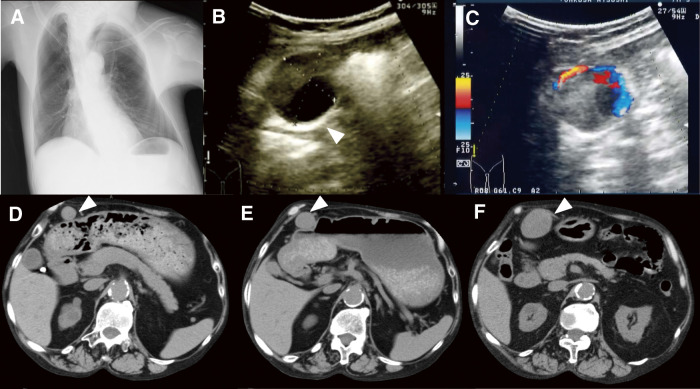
Fig. 1 Image findings. A chest X-ray revealed a scoliosis deformity (A). A sagittal sonogram identified a large anechoic mass (arrowhead) in the right hypochondrium (B). Color Doppler sonography demonstrated blood flow within the mass (C). Computed tomography imaging showed progressive enlargement of the gastroepiploic artery aneurysm, with its maximum diameter measuring 18 mm one year prior (D), increasing to 26 mm five months before admission (E), and expanding to 50 mm at the time of admission (F).
